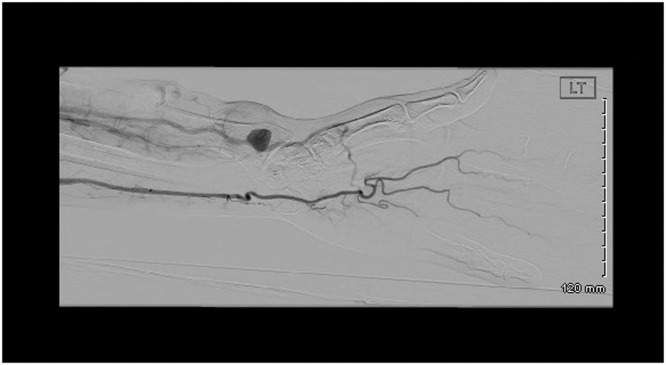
CT angiogram showing an aneurysmal dilatation of the radial artery and poor perfusion of the radial palmar arch with intact ulnar palmar arch.
